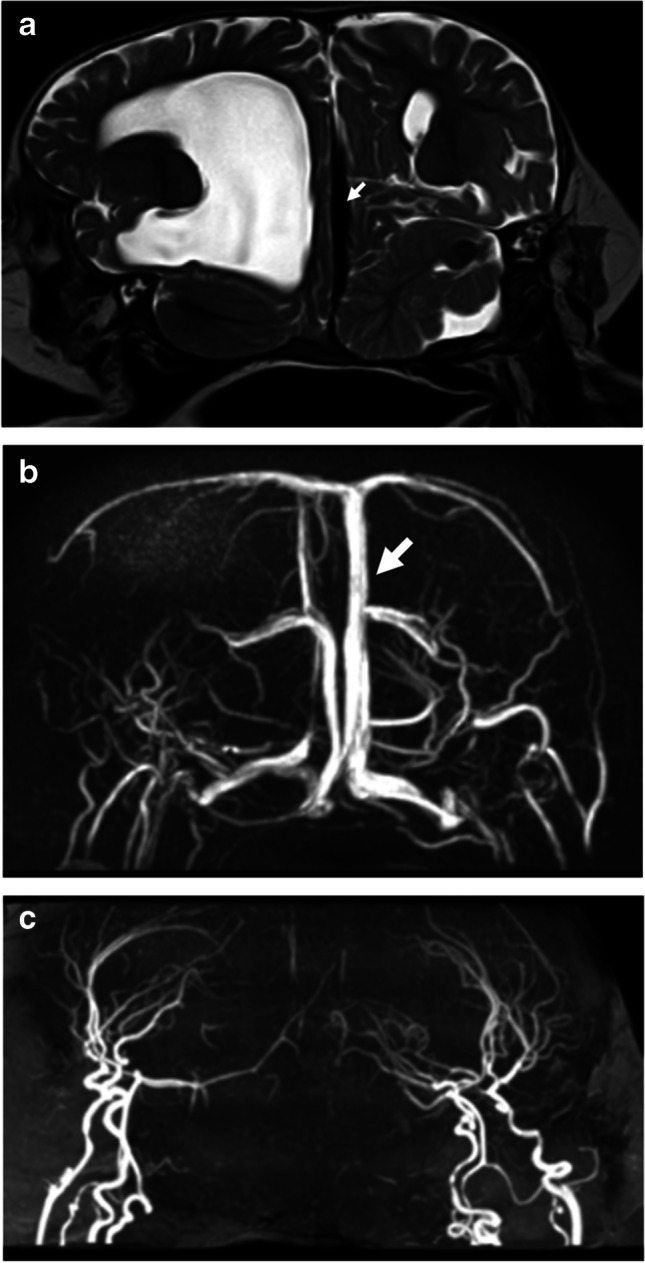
Magnetic resonance images in 2-year-old craniopagus conjoined twin girls. a Coronal T2-weighted image shows cranial fusion with two separate brains with an intervening common venous sinus (*arrow*). b Coronal magnetic resonance venography image confirms sharing of the superior sagittal sinus (*arrow*). c Coronal magnetic resonance arteriography image reveals no significant arterial sharing
